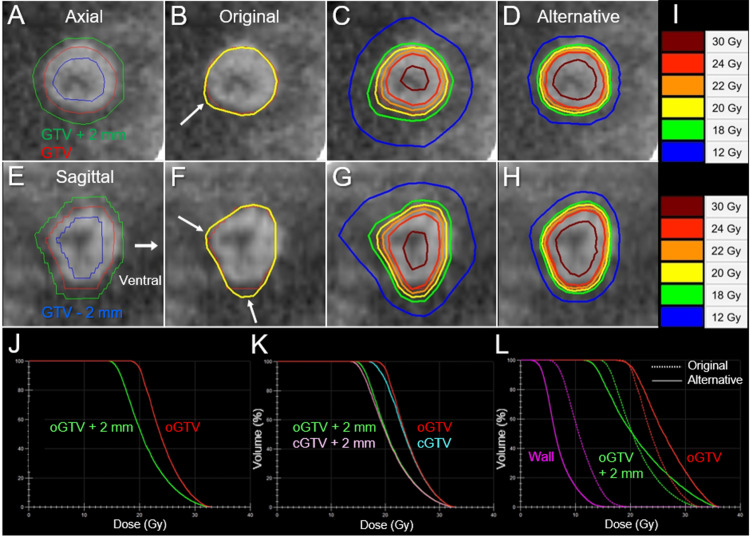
Target definition, dose distributions, and dose-volume histograms. The images show CECT images for SRS planning (A-H); target definition (A, E); dose distributions for the original (B, C, F, G) and alternative plans (D, H); axial images (A-D); sagittal images (arrow in E indicating the ventral side) (E-H); representative isodoses (I); and dose-volume histograms (DVHs) (J-L). (A, E) The original GTV and uniform 2-mm added and reduced objects (GTV + 2 mm, GTV – 2 mm). The contours of margin-added or reduced structures generated on Monaco® (Elekta AB, Stockholm, Sweden) are not smooth but slightly jerky. (B, F) The 20 Gy isodose lines covers 95% of the original GTV (oGTV) with some over-coverage (arrows in B, F). (D, H) The dose gradients just outside and inside the oGTV boundary in the alternative plan (D, H) are steeper and more concentrically laminated than those for the original plan (C, G). (J) The DVHs for the oGTV and oGTV + 2 mm. (K) The DVHs for the corrected GTV (cGTV) and cGTV + 2 mm in addition to the oGTV and oGTV + 2 mm. (L) Comparison of the DVHs for the original and alternative plan. The wall structure is the 8-mm thickness object outside the oGTV + 2 mm boundary. CECT: contrast-enhanced computed tomography; SRS: stereotactic radiosurgery; GTV: gross tumor volume
